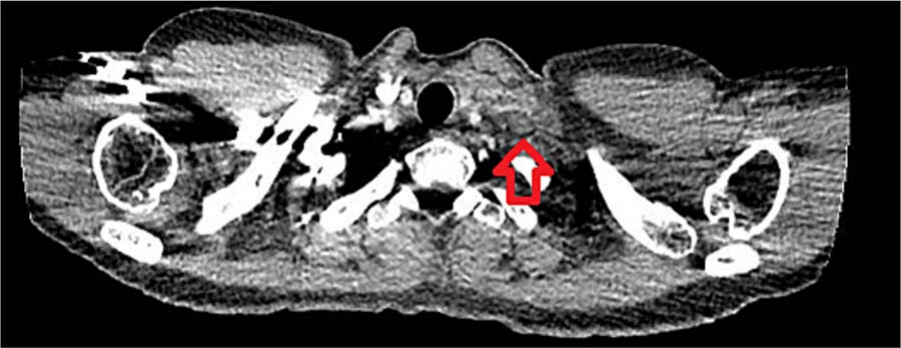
CT thorax showed a 25 × 9 mm supraclavicular mass.
